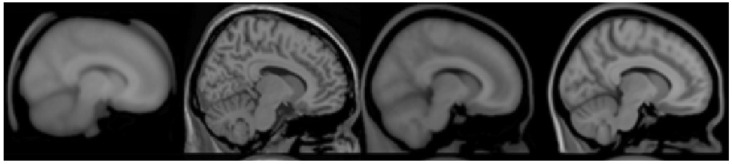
The sagittal plane of standard MRI templates: average305, colin27, ICBM152 T1, NLICBM152 T1 (from left to right).
